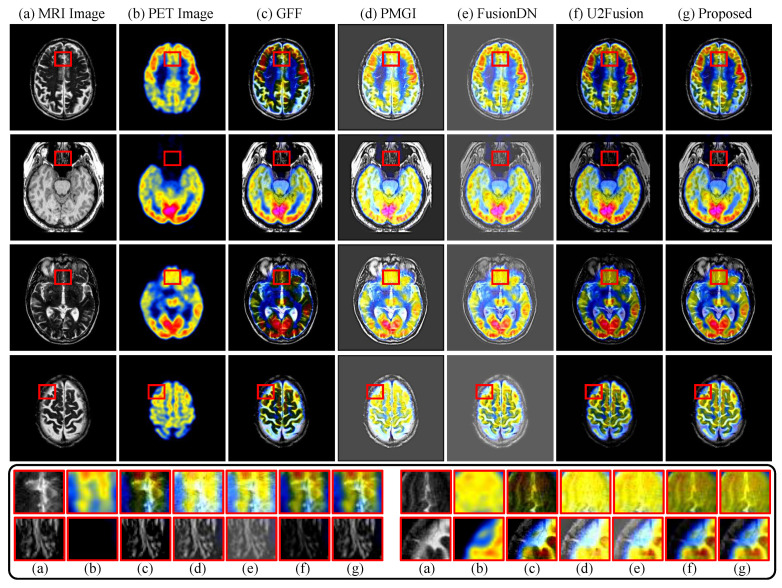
Fusion results for PET/MRI images on Havard dataset of our proposed method and four image fusion methods: GFF [45], PMGI [46], FusionDN [33] and U2Fusion [8]. The zoom-in patches of corresponding images (a–g) are shown in the black box.
